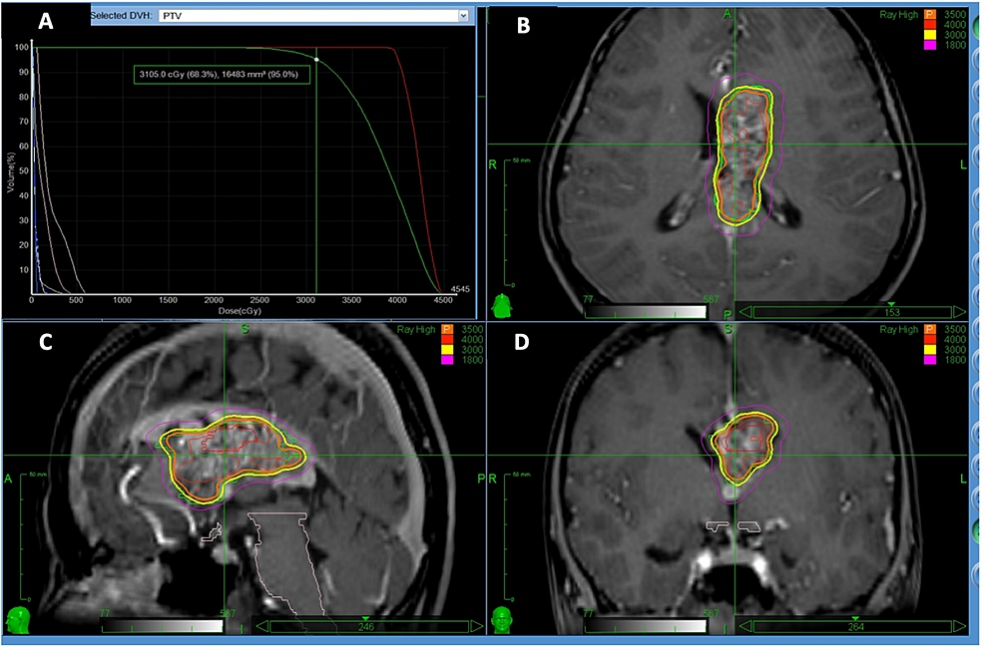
Dose-planning of stereotactic radiotherapy Through CyberKnife M6, the AVM with a GTV of 17.3 ml received 35 Gy in five fractions, with an isodose line of 77%. Planned target volume (orange) covered by the 77% isodose can be seen in axial (B), sagittal (C), and coronal (D) planes on pretreatment planning computed tomography angiography (CTA). The upper left graph (A) shows that 95% of the area was irradiated with more than 31 Gy. The maximum dose was 45 Gy.
